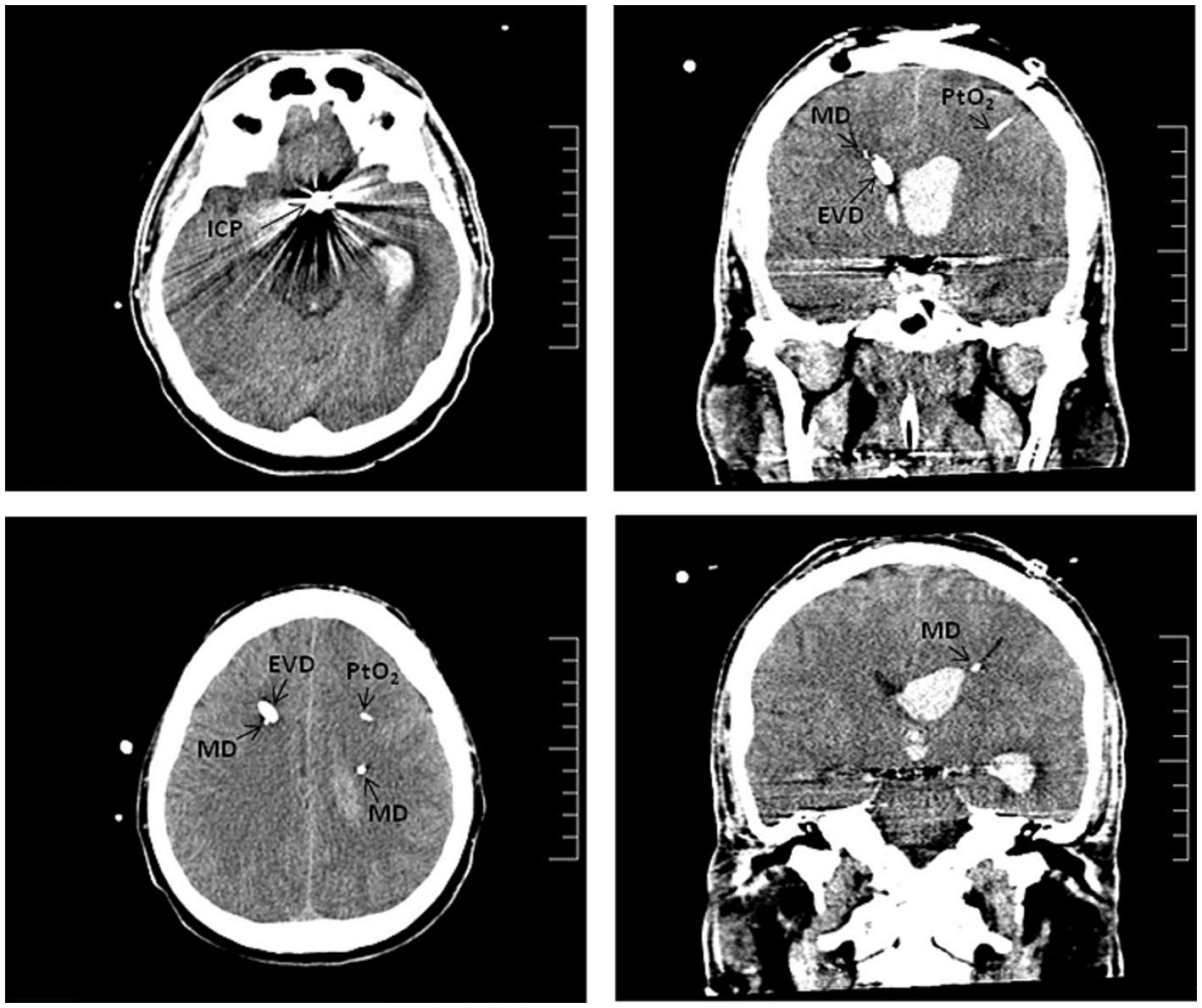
Computed tomography scan of aSAH-patient with poor initial clinical status. SAH due to rupture of an aneurysm in the left ACom. Axial (left panels) and coronal (right panels) sections show the ICP sensors, the catheters for bilateral microdialysis monitoring (MD), the external ventricular drain (EVD) and the PtO2 sensor.
